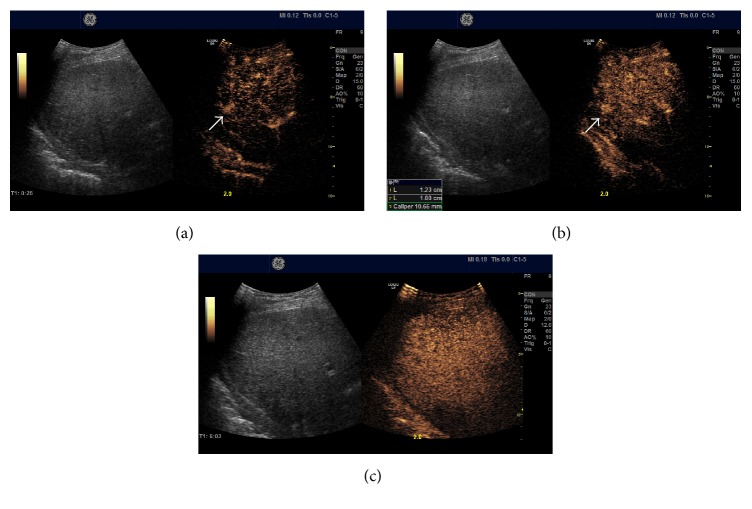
Small HCC (10 mm) nonvisible on B mode ultrasound but was hyperenhanced during arterial phase (a) and portal venous (b) phase. It was slightly hypoenhanced in the late phase (c) on contrast-enhanced ultrasound. The arrow in (a) and (b) refers to the hyperenhanced small HCC lesion.
